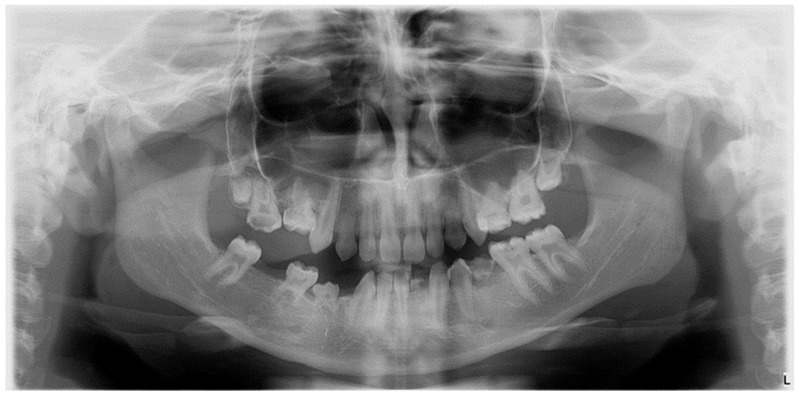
Radiographic image of developmental dental anomalies (number, size, structure).
